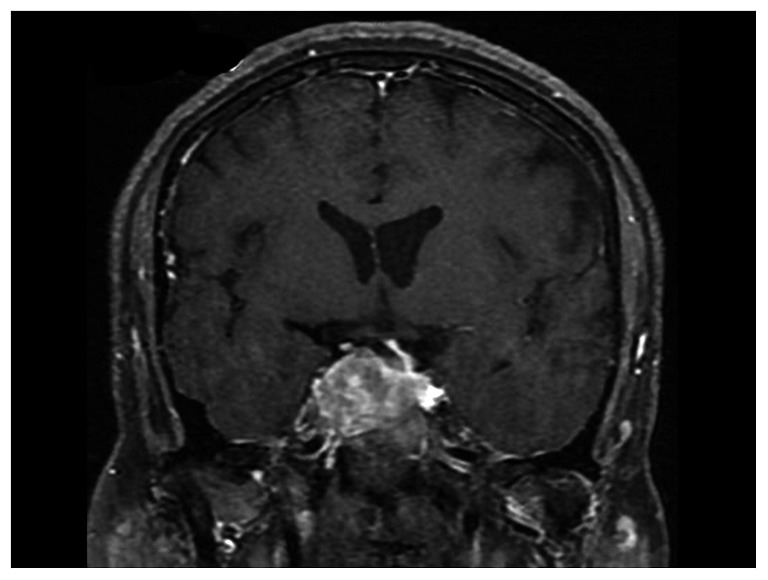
Cranial coronal T1‐weighted MRI with contrast enhancement showed a sellar irregular lesion with a leftward deflection of the hypophysis stalk
